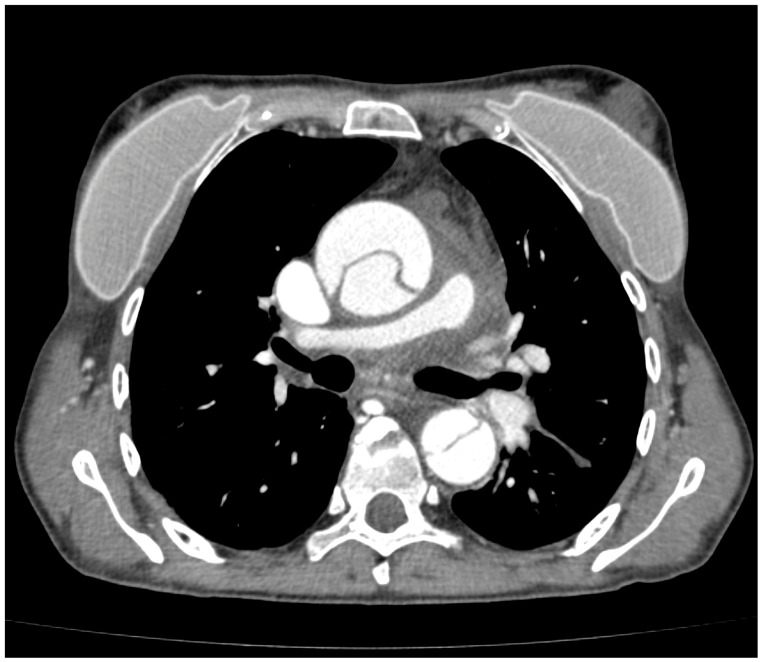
CT angiography demonstrating extensive Stanford type A aortic dissection extending from the ascending aorta to the iliac arteries, with branch vessel involvement and associated pericardial effusion. These findings reflect severe aortic wall instability with systemic and visceral complications.
